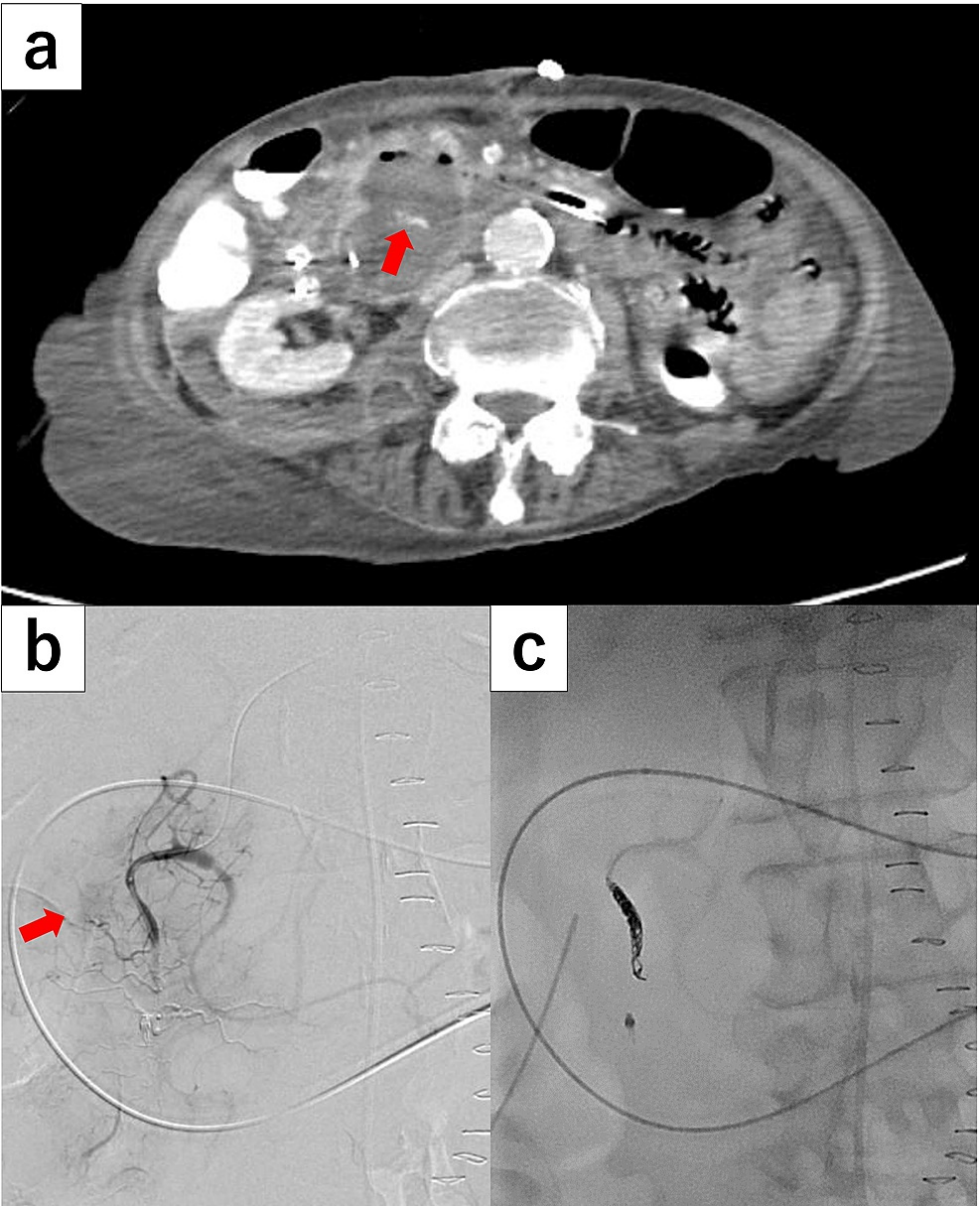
Computed tomography and emergency angiography. (a) CT scan showing extravasation (red arrow) from the neck of the duodenal diverticulum (as seen previously). (b) Emergency angiography showing extravasation (red arrow) from a distal branch of the anterior superior pancreaticoduodenal artery. (c) Hemostasis is achieved by embolization.
